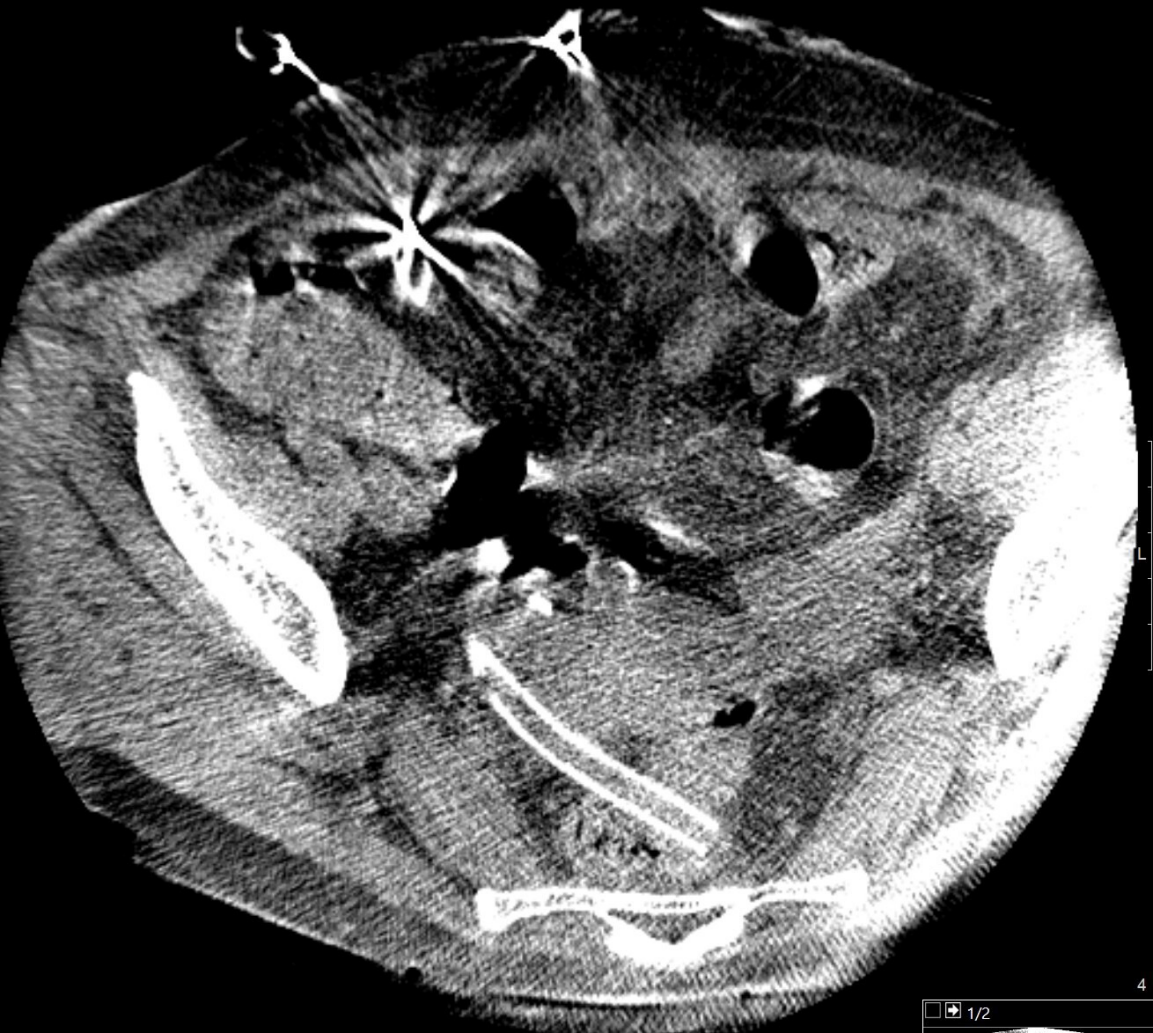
Cone beam CT identifying drain position and no evidence of kinking
